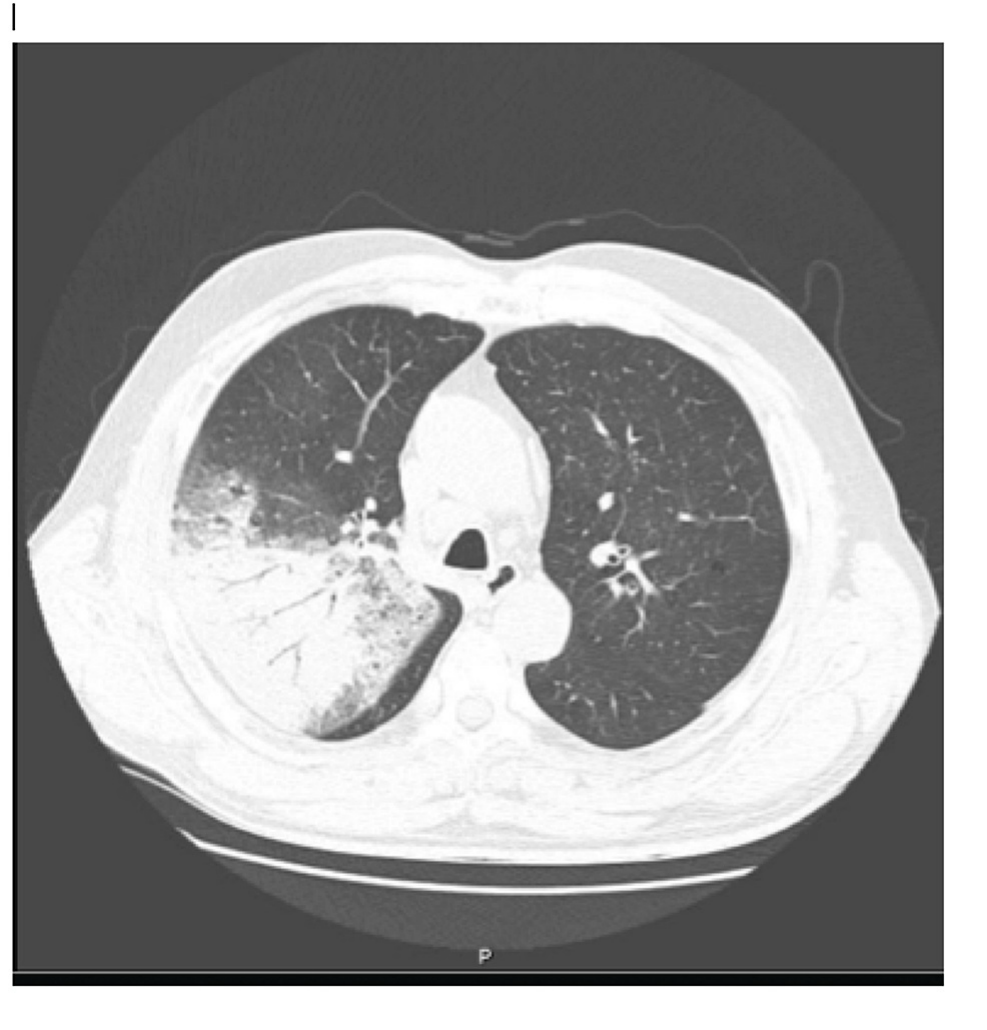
Thoracic computed tomography in the upper apex.
